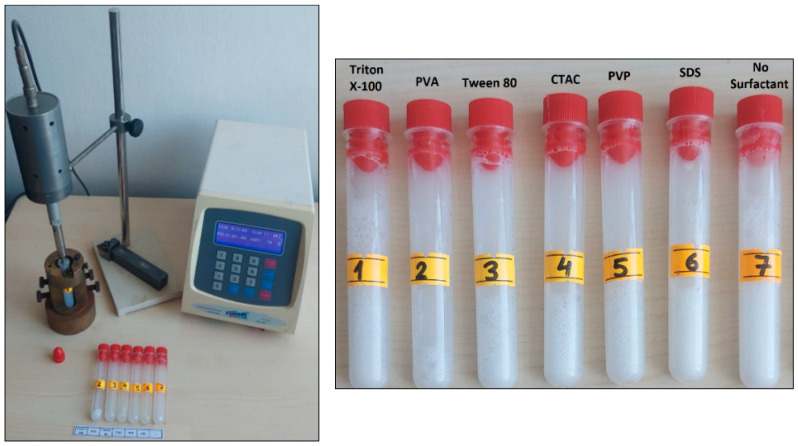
Prepared nanofluids using an ultrasonic homogenizer.
